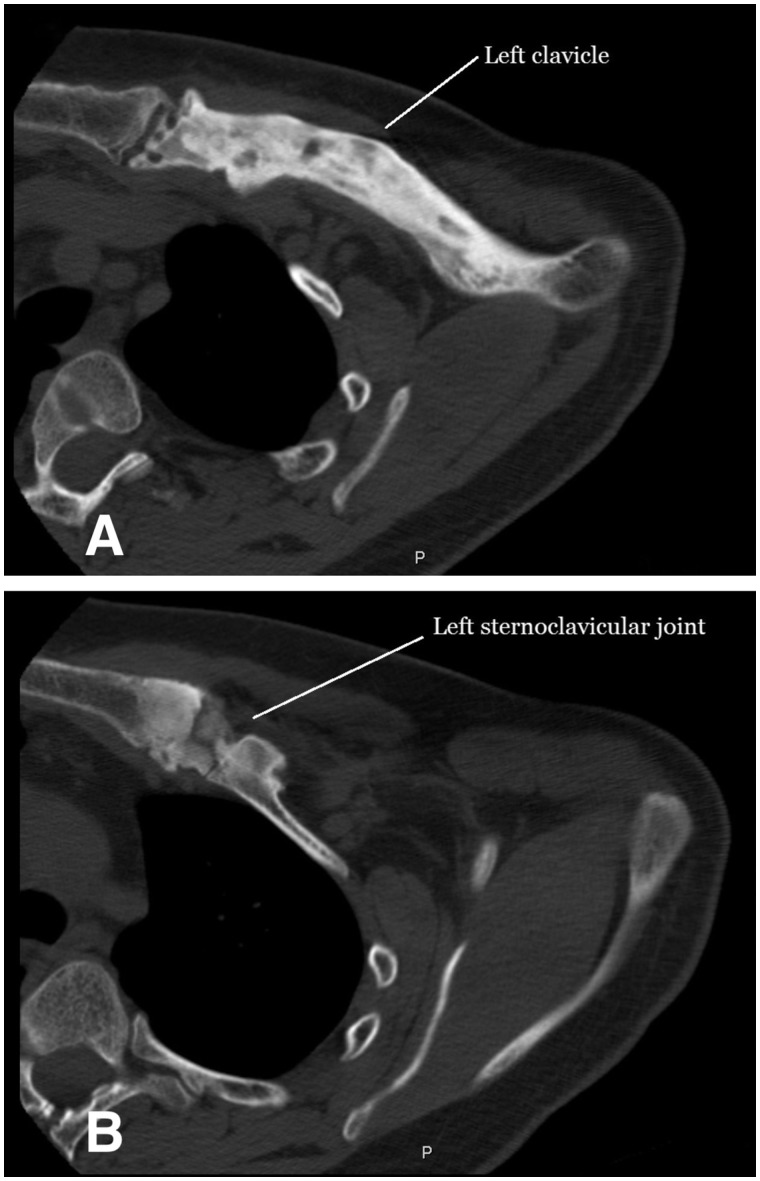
CT of the left clavicle (A) Osteosclerosis and bone expansion of the left clavicle extending to the sternoclavicular joint. (B) Osteitis and hyperostosis of the left sternoclavicular joint.
